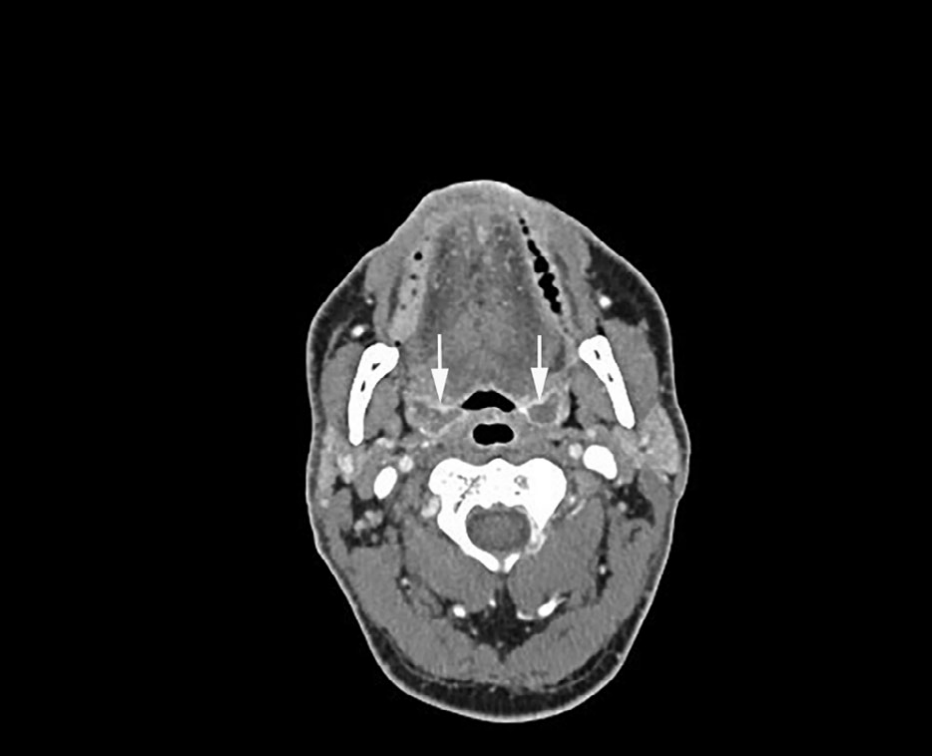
Head and neck computed tomography (CT) revealed involvement of the bilateral palatine tonsils (white arrows).
